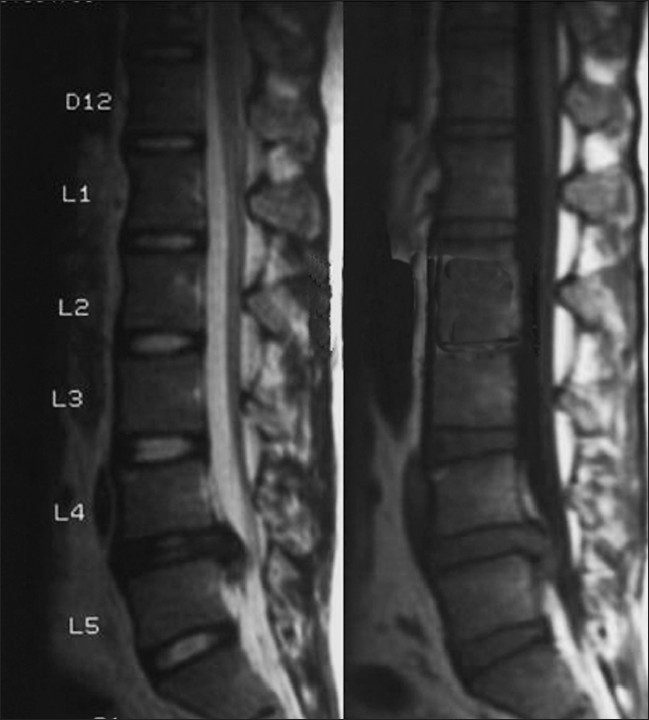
Sagittal section (T1 and T2 weighted) MRI showing herniated disc at L4-5 level
